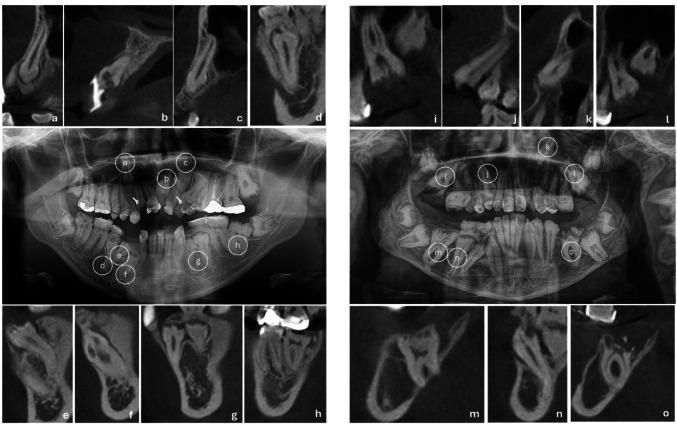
Panoramic radiographs and CBCT sagittal slices of patient 1 a–h and patient 2 i–o to show the PECR in impacted teeth. Patient 1 presented nine teeth with PECR (a to h), and patient 2 presented seven teeth with PECR i–o. In all the sagittal slices, note the irregular coronary crown surface in different degrees of severity. Some teeth presented a more severe PECR (b, f, i, k, m, and o). Enlarged dental follicles were observed in some teeth of Patient 1 a, d,e, f,g, and h) and Patient 2 i, l,m, n, and o. Adjacent impacted teeth presented fused dental follicles g, i, l, and n
